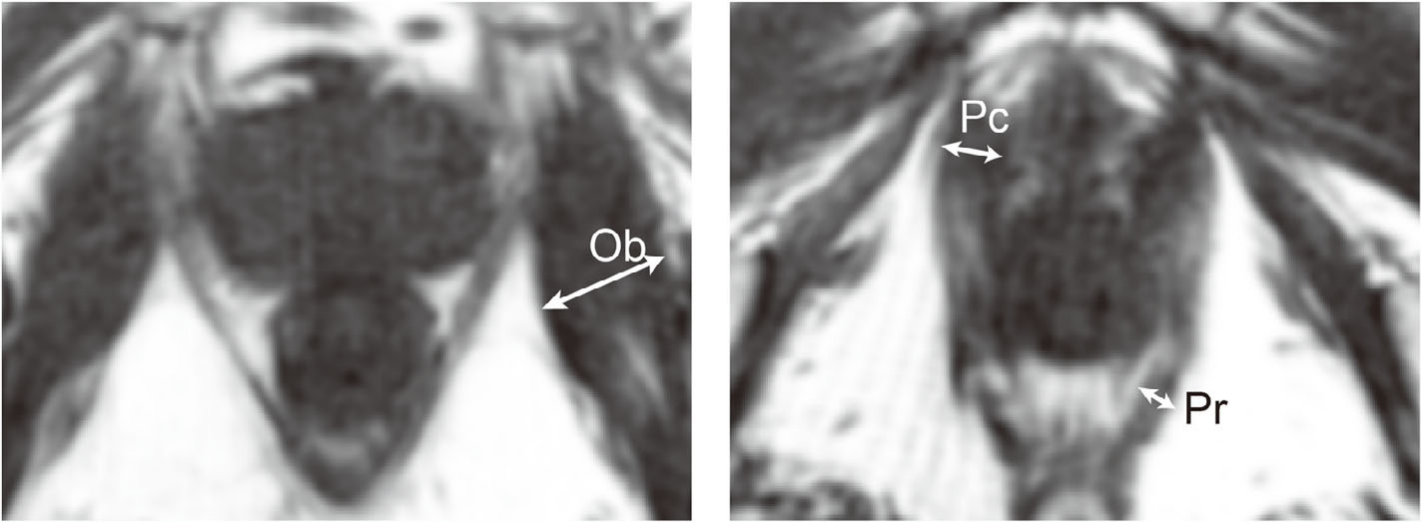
Measurement of the maximum diameter (indicated by arrows) of pelvic floor muscles on magnetic resonance imaging (MRI). Measurements were conducted in triplicates for each muscle to ensure precision. Ob, obturator internus muscle; Pc, pubococcygeus muscle; Pr, puborectalis muscle.
